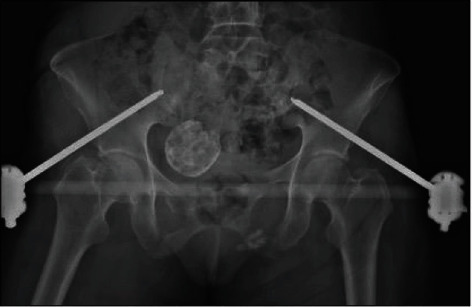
Anteroposterior pelvis radiograph showing the external fixator in place and the antibiotic beads surrounding the left inferior pubic rami fracture.
